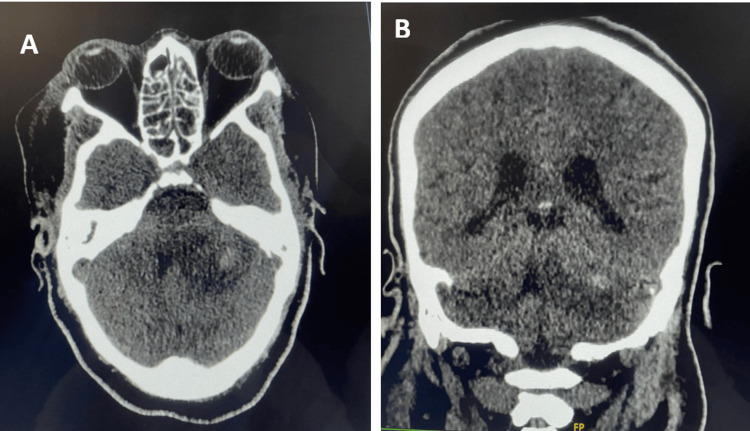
Follow-up brain CT. Images are showing liquefaction of the brainstem hematoma on (A) axial images and (B) coronal reconstruction.
